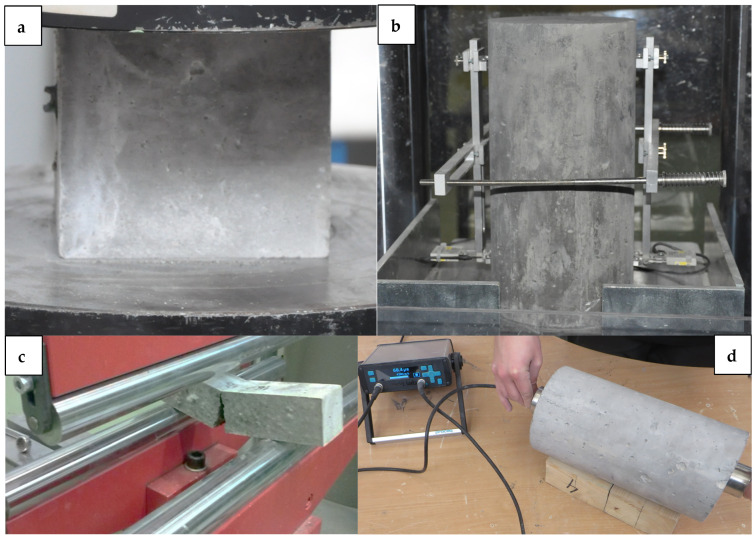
Tests of HPC samples: (a) compressive strength, (b) modulus of elasticity, (c) flexural strength, and (d) ultrasonic pulse velocity.
